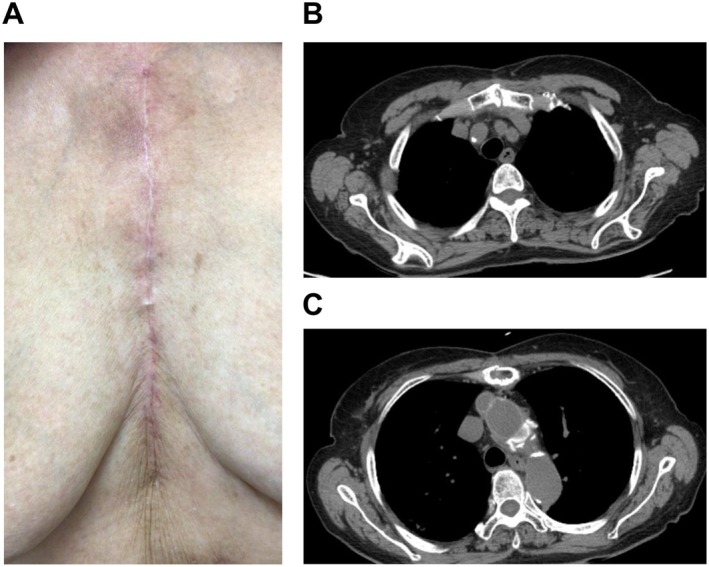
Postoperative course. (A) Skin findings 3 months postoperatively: No abnormalities were observed. (B) Computed tomography 6 months postoperatively showing no displacement of the manubrium. (C) No displacement of the sternal body.
